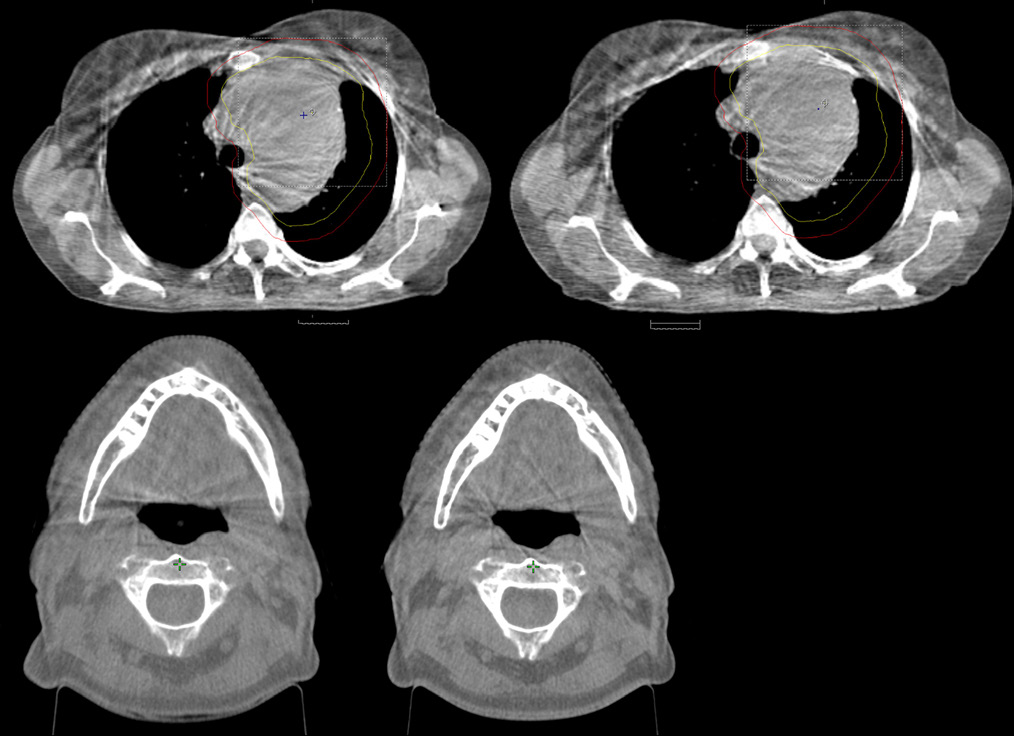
Thoracic scan taken with Varian default pelvis CBCT protocol (top left) and scan taken with Thorax_S (top right). Head scan taken with Varian default Head CBCT protocol (bottom left) and scan taken with Head_Standard (bottom right). Both Thorax_S and Head_Standard decreased the imaging dose without affecting the radiographers’ confidence to perform online image registration.
